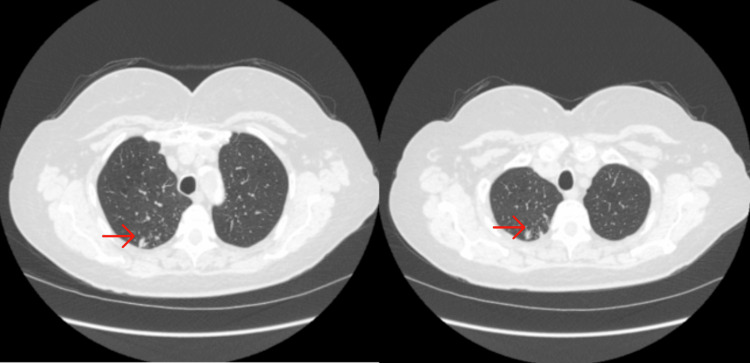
CT chest performed three months prior to the initial presentation to the Outpatient Department of East Carolina University Pulmonology Clinic demonstrating multiple RUL and RML nodular opacities. RUL: right upper lobe; RML: right middle lobe
